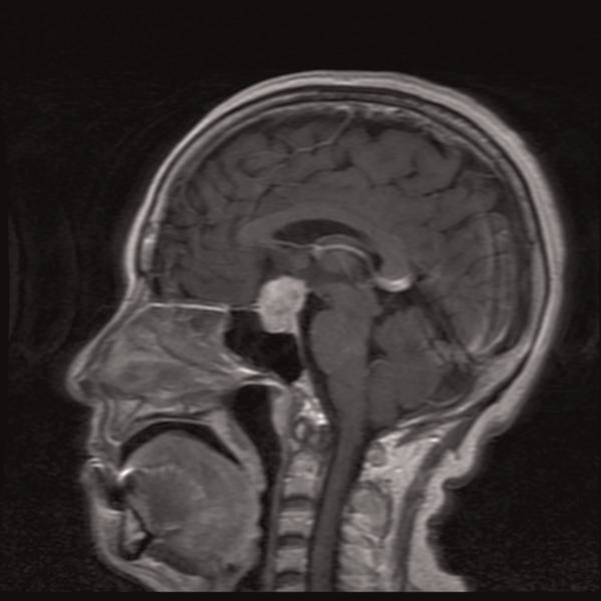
Gadolinium-enhanced sagittal MRI showing an expanding lesion inside the sella with suprasellar extension.
